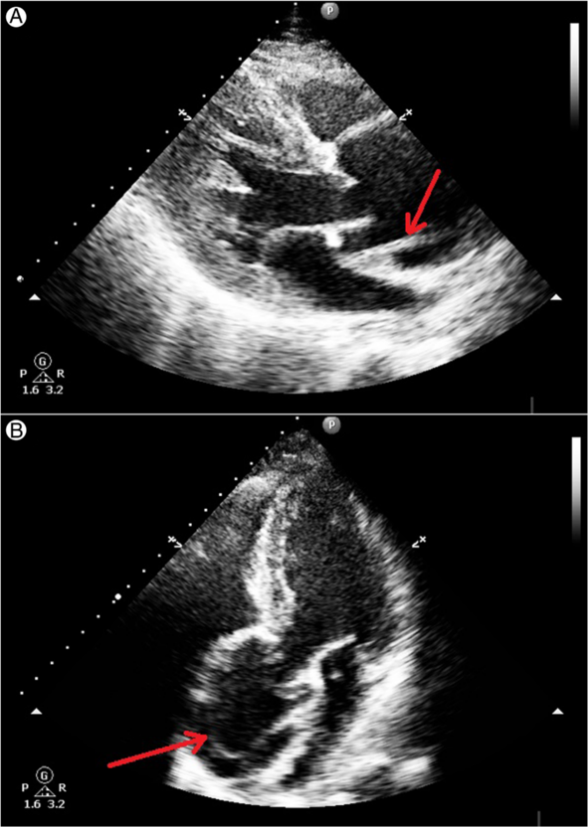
Transthoracic echocardiography showing linear echo of intimal tear in dilated aortic root above aortic valve level. A. Transthoracic echocardiography – parasternal long axis view: linear echo of intimal tear is seen just above aortic valve in systole. B. Transthoracic echocardiography – apical 5-chamber view: linear echo of intimal flap is visible in dilated aortic root above aortic valve level.
